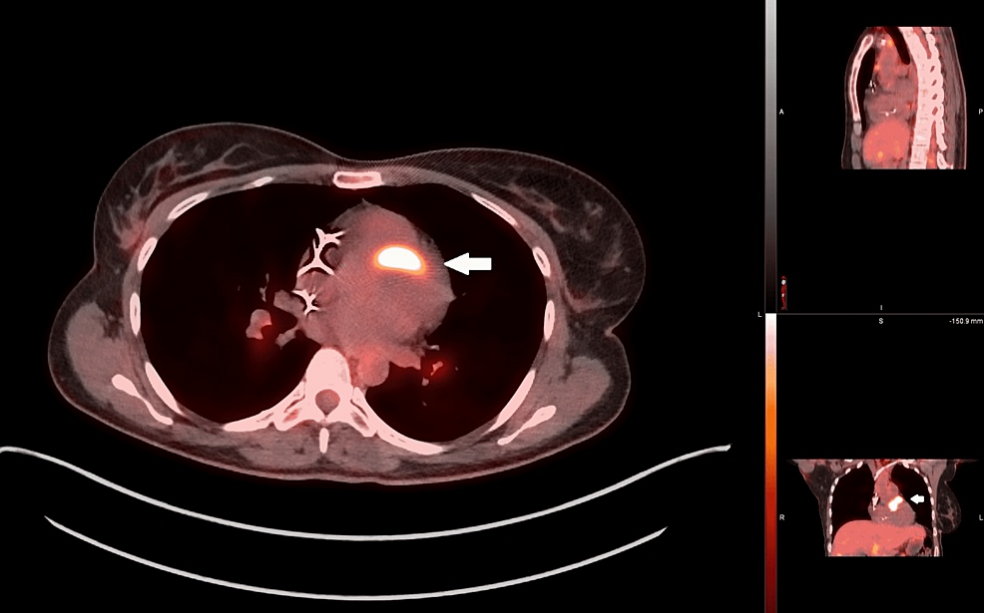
Cardiac PET scan reveals FDG avidity in the anteroseptal region of the myocardium FDG: fluorodeoxyglucose; PET: positron emission tomography.
